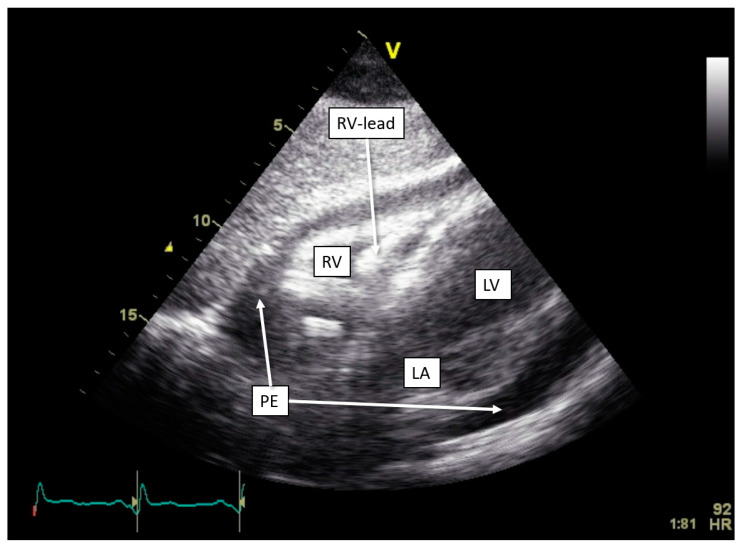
Development of pericardial effusion in OptiVol (Medtronic) alert. Transthoracic echocardiogram with subcostal view, showing pericardial effusion measuring approximately 20 mm circumferentially. Abbreviations: LA = left atrium, LV = left ventricle, PE = pericardial effusion, RV = right ventricle.
